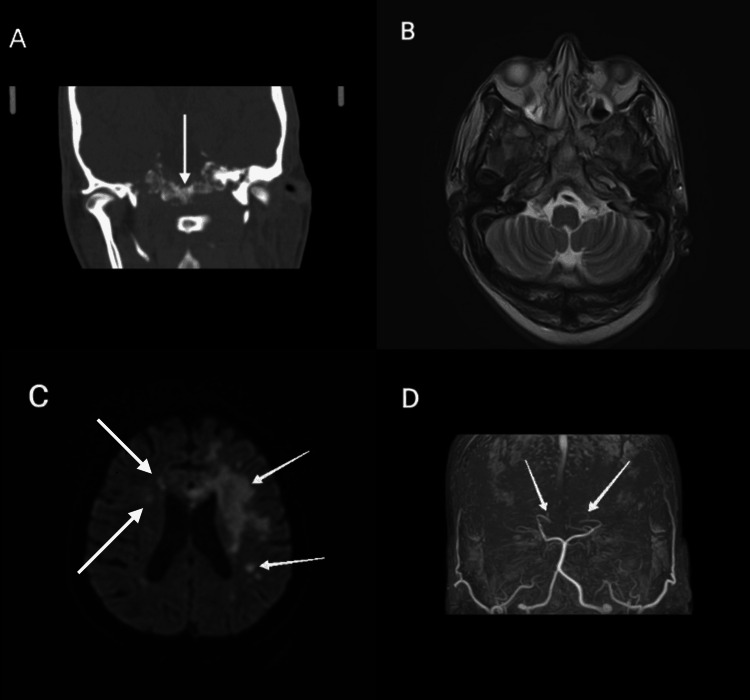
A (coronal CT head bone window) and B (MRI brain axial T2-weighted image) showing osteopenia and altered signals along the base of the skull, i.e., body and greater wings of the sphenoid bone. C (MRI brain axial DWI) showing significant diffusion restriction in the left fronto-parietal, medial temporal, capsuloganglionic regions and the genu of the corpus callosum suggestive of acute infarcts, in addition to multiple small right-sided acute infarcts. D (MR cerebral angiogram) showing non-visualization of bilateral ICAs and anterior circulation, suggestive of thrombosis
